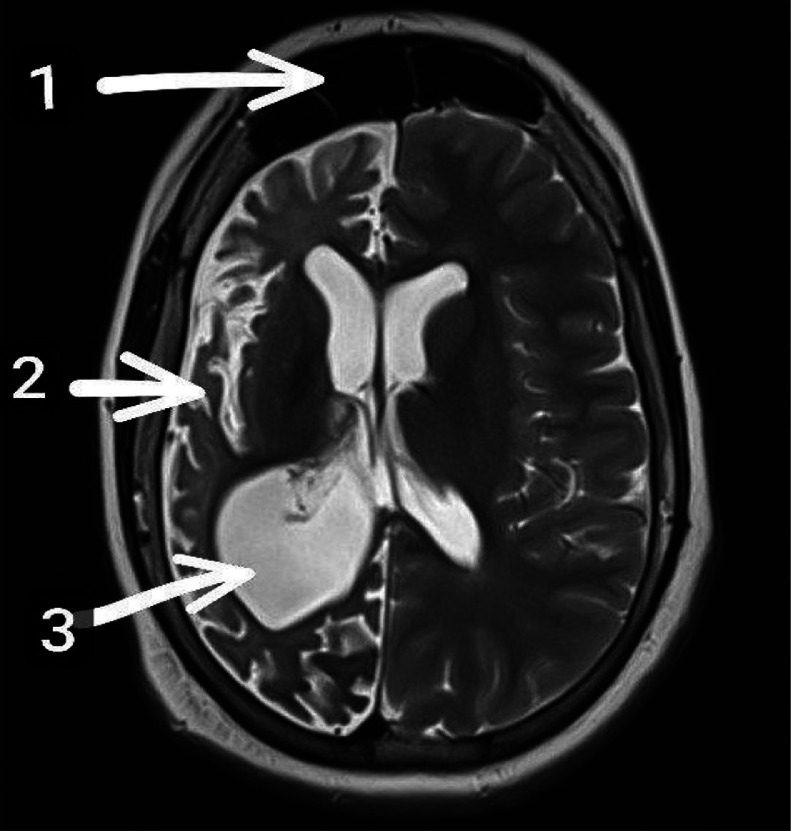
Axial T2-weighted MRI of the brain shows characteristic features of Dyke-Davidoff-Masson syndrome. Note the hyperpneumatization of the right frontal sinus (1), atrophy of the right cerebral hemisphere (2), and ipsilateral (right) ventriculomegaly (3). No contrast-enhanced MRI was performed. 1. Hyperpneumatization of the right frontal sinus. 2. Right cerebral hemisphere atrophy. 3. Ipsilateral (right) ventriculomegaly.
